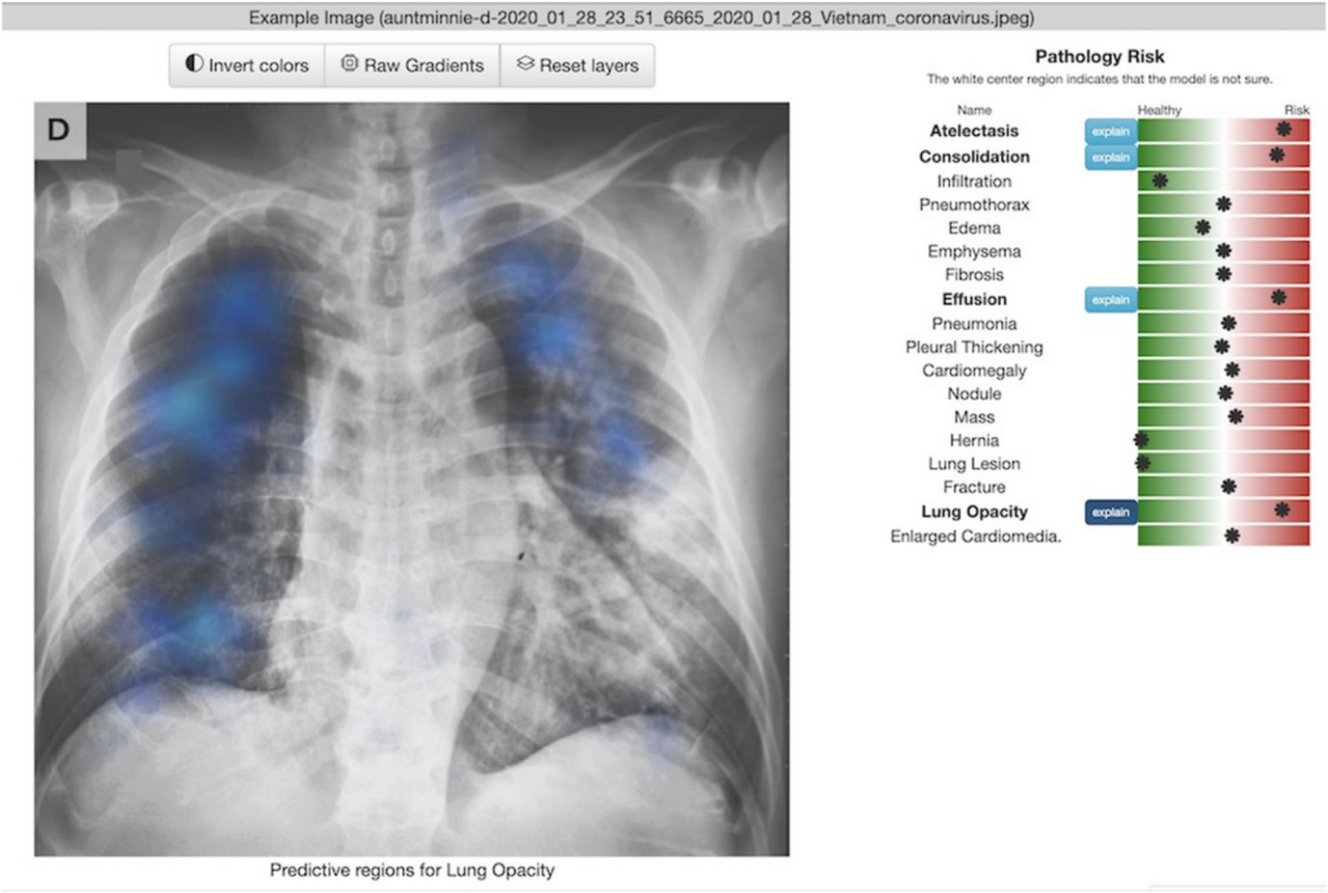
Chester AI tool, showing pathology risk scores and a saliency map for predictive Lung Opacity regions on a CXR. Image is used under fair use from open website “Chester The AI Radiology Assistant” (https://mlmed.org/tools/xray/).
